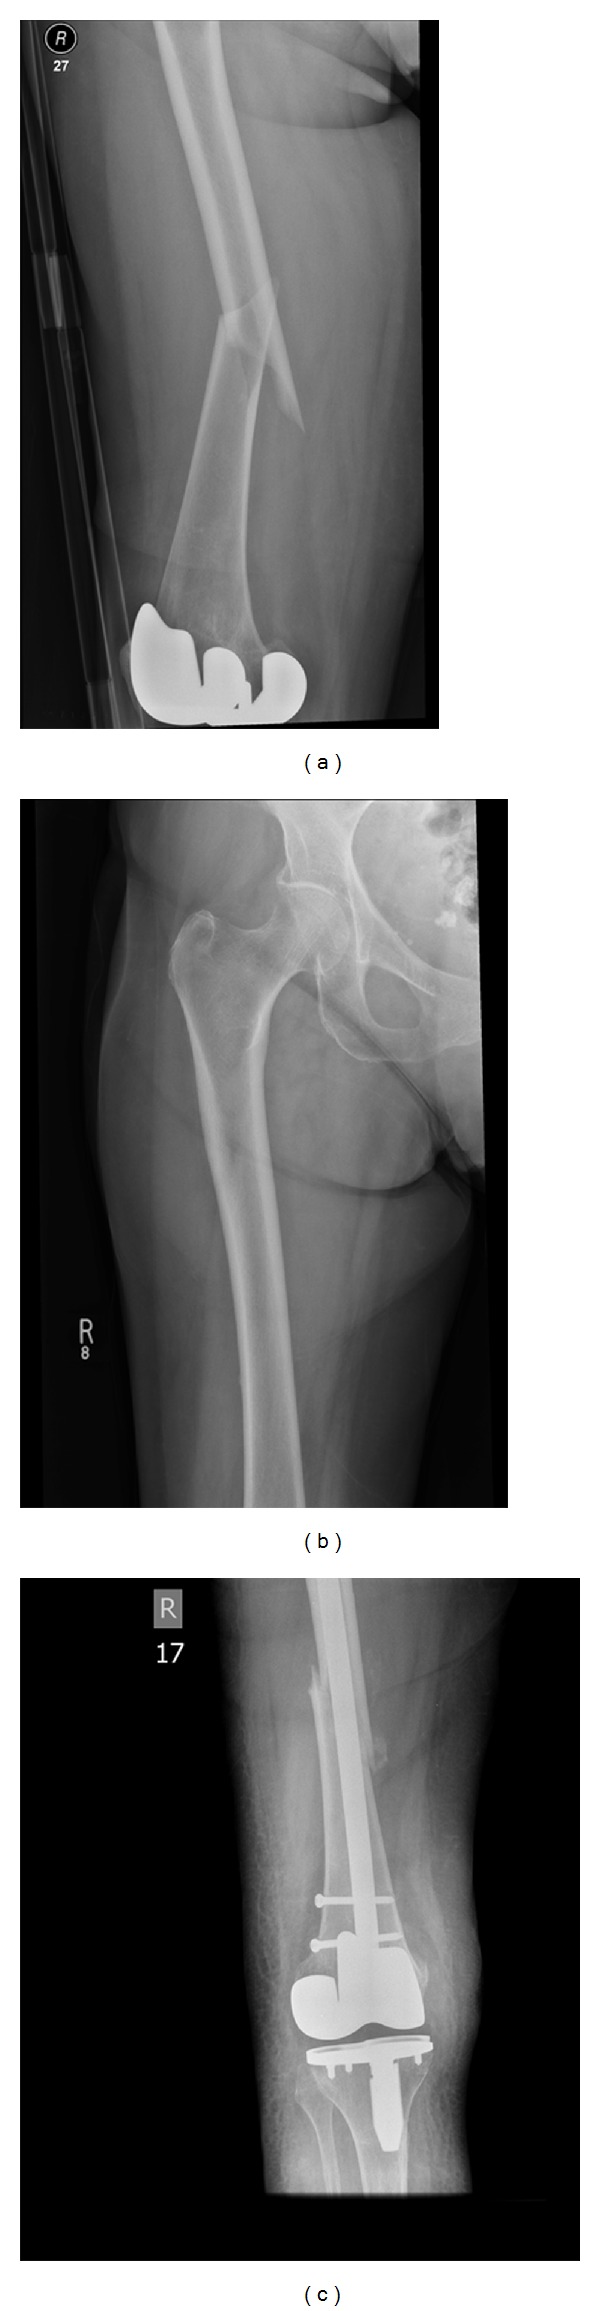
(a) Admission radiograph showing noncomminuted fracture with medial spike. (b) Radiograph taken 4 weeks prior to admission revealing subtle periosteal reaction on lateral cortex. (c) Radiograph 12 days postoperative repair.
